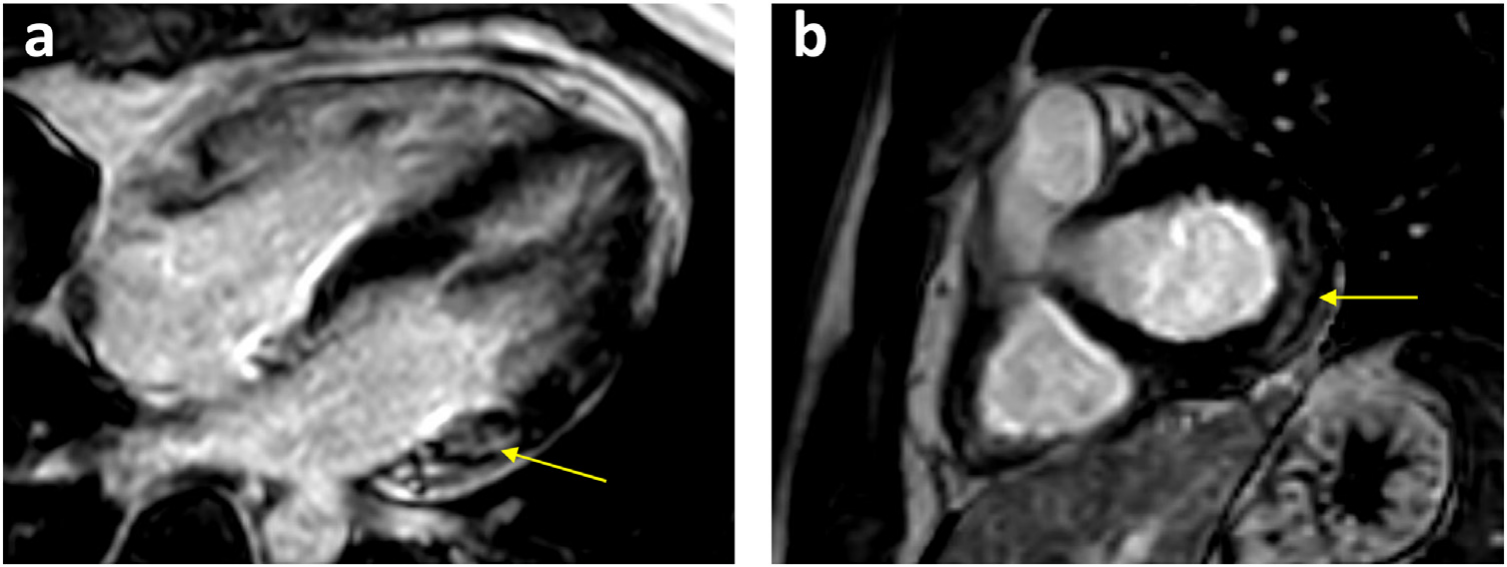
Cardiac MRI PSIR– LGE views showing late gadolinium subepicardial enhancement in basal lateral segments in both (a) Four Chamber and (b) Short Axis views.
